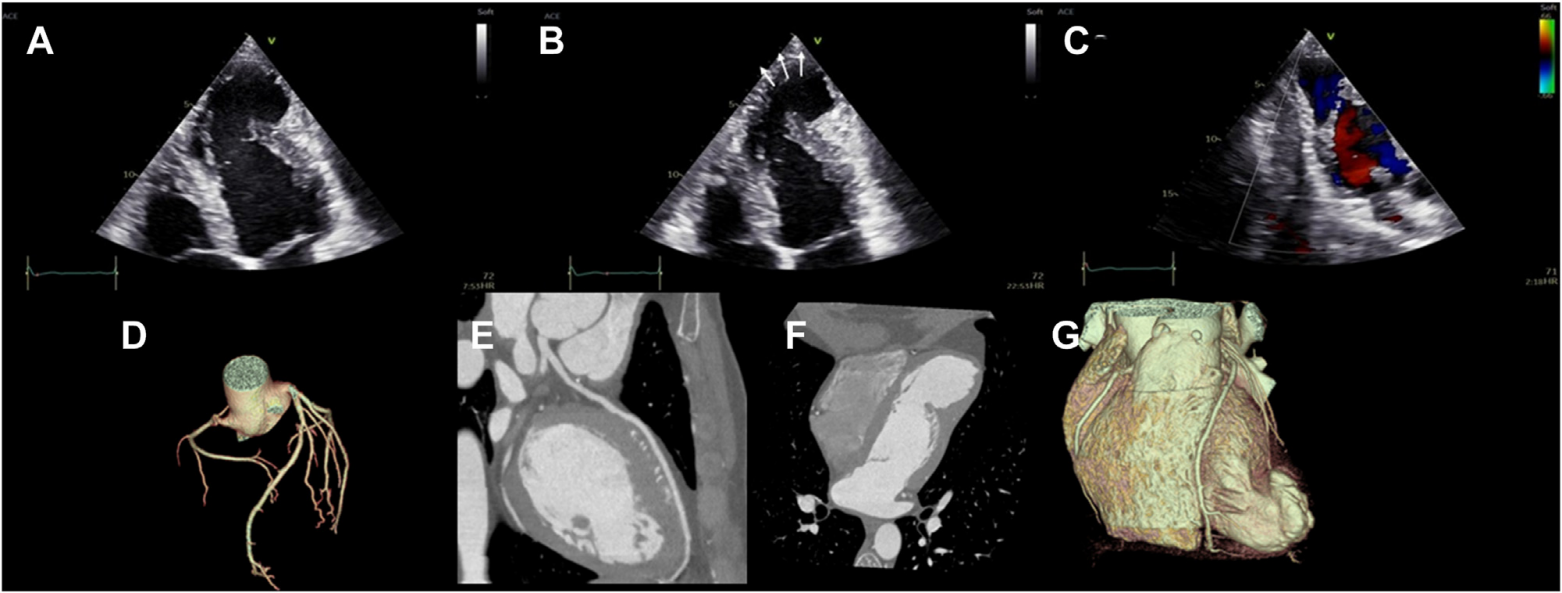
Transthoracic echocardiography and cardiac computed tomography. Long-axis 4-chamber view of the (A) diastolic frame and (B) systolic frame showing a left ventricular (LV) apical aneurysm with a wide neck connecting it to the LV cavity. Arrows indicate dyskinetic wall motion (systolic paradoxical expansion). (C) Color Doppler imaging shows no flow acceleration across the aneurysmal neck. (D) Computed tomography coronary 3-dimensional reconstruction showing normal origin and course of epicardial coronary arteries. (E) Absence of left anterior descending coronary artery stenosis is demonstrated. (F) Reformatted apical view and (G) volume-rendered 3-dimensional reconstruction showing the LV aneurysm.
